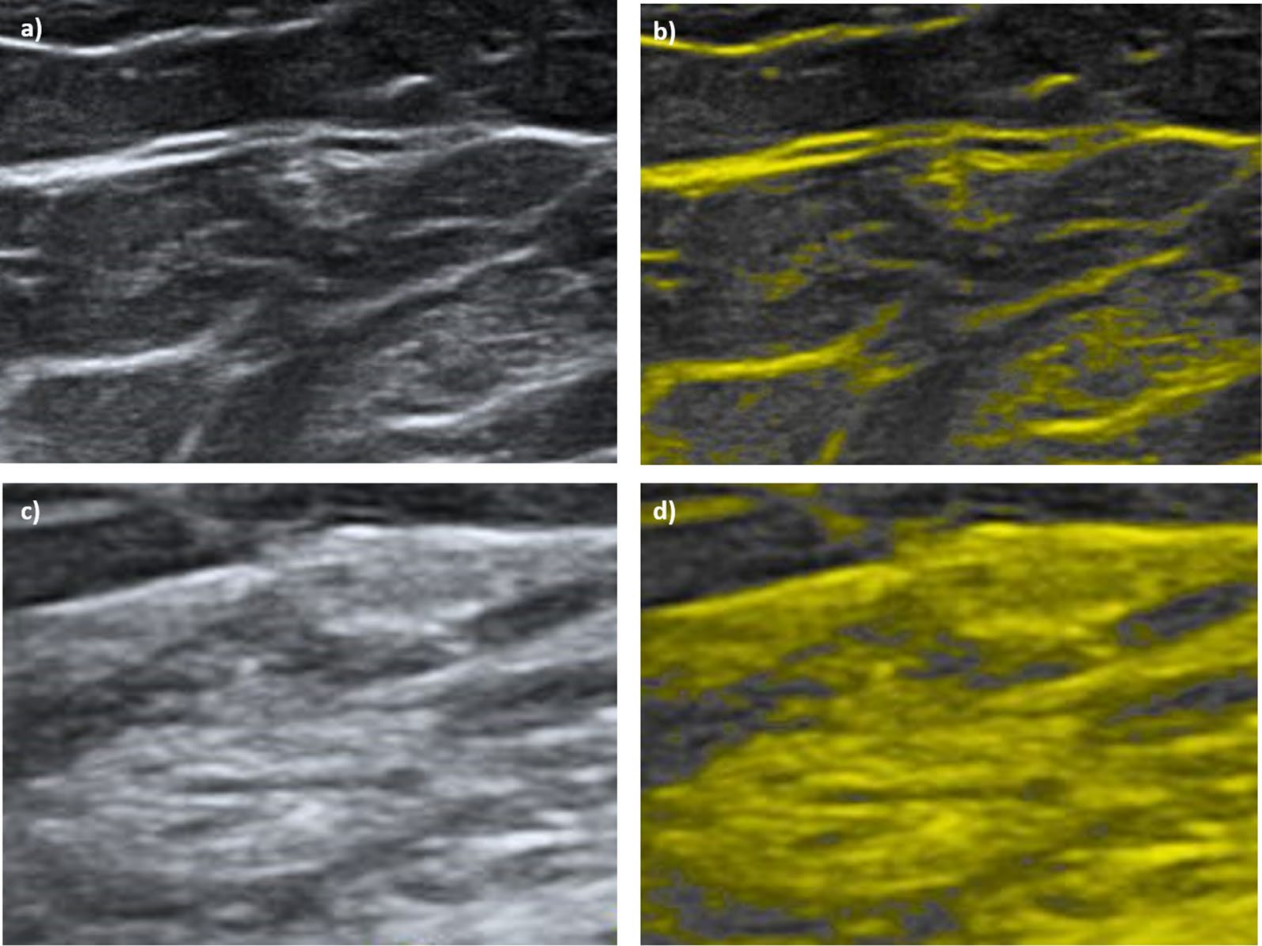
Example of ultrasound images for one patient with low mammographic density (a, b) and one patient with high mammographic density (c, d). Dotted lines refer to the pectoralis muscle. Colored markings indicate areas with high breast density. For better visualization, the Madena software was used. a The original ultrasound image of the left breast, upper outer quadrant for a patient with low breast density, indicated by only small colored areas in b. c The original ultrasound image of the left breast, upper outer quadrant for a patient with high breast density indicated by larger colored areas in d
